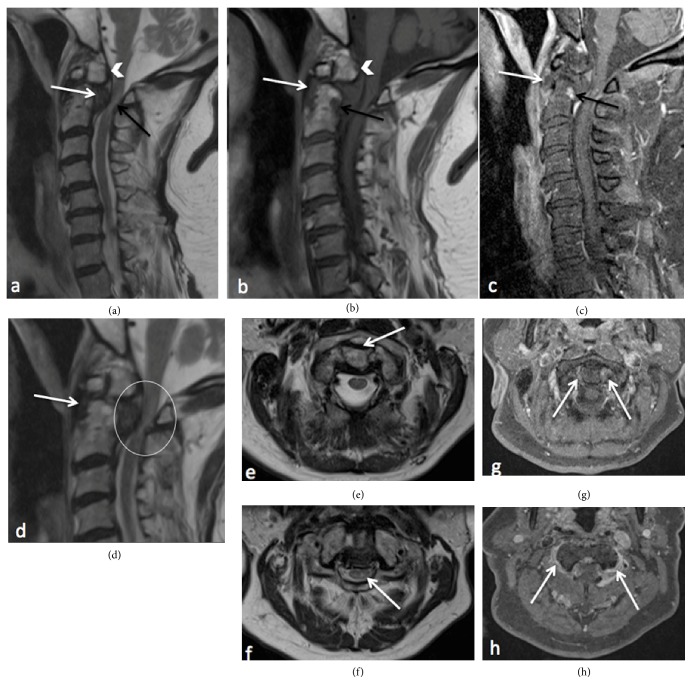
Complementary cervical enhanced MRI with Gadolinium administration, acquired to investigate the cervical spinal cord compression, showing the ROP as low signal intensity on both T1w and T2w images in sagittal planes surrounding the body of C2 and this ROP (a,b,d, white arrows). It appears without enhancement on T1w fat sat images after gadolinium administration in sagittal (c, white arrow) and axial (g, white arrows) planes. We can see some enhancement on axial T1w fat sat after contrast administration around interfacetar articulations of C1-C2 (h, white arrows), probably from degenerative origin. A sub centimetric geode in the basis of C2 shows low signal on T1w image in sagittal plane (b, black arrow) with an enhancement after contrast administration in axial plane (c, black arrow). The T2w image in sagittal plane reveals an area of high intensity in the intramedullary regions of C1 and C2 (d, white circle), due to compression by the ROP, but without diastasis between anterior arch of C1 and the dens (e, white arrow).
